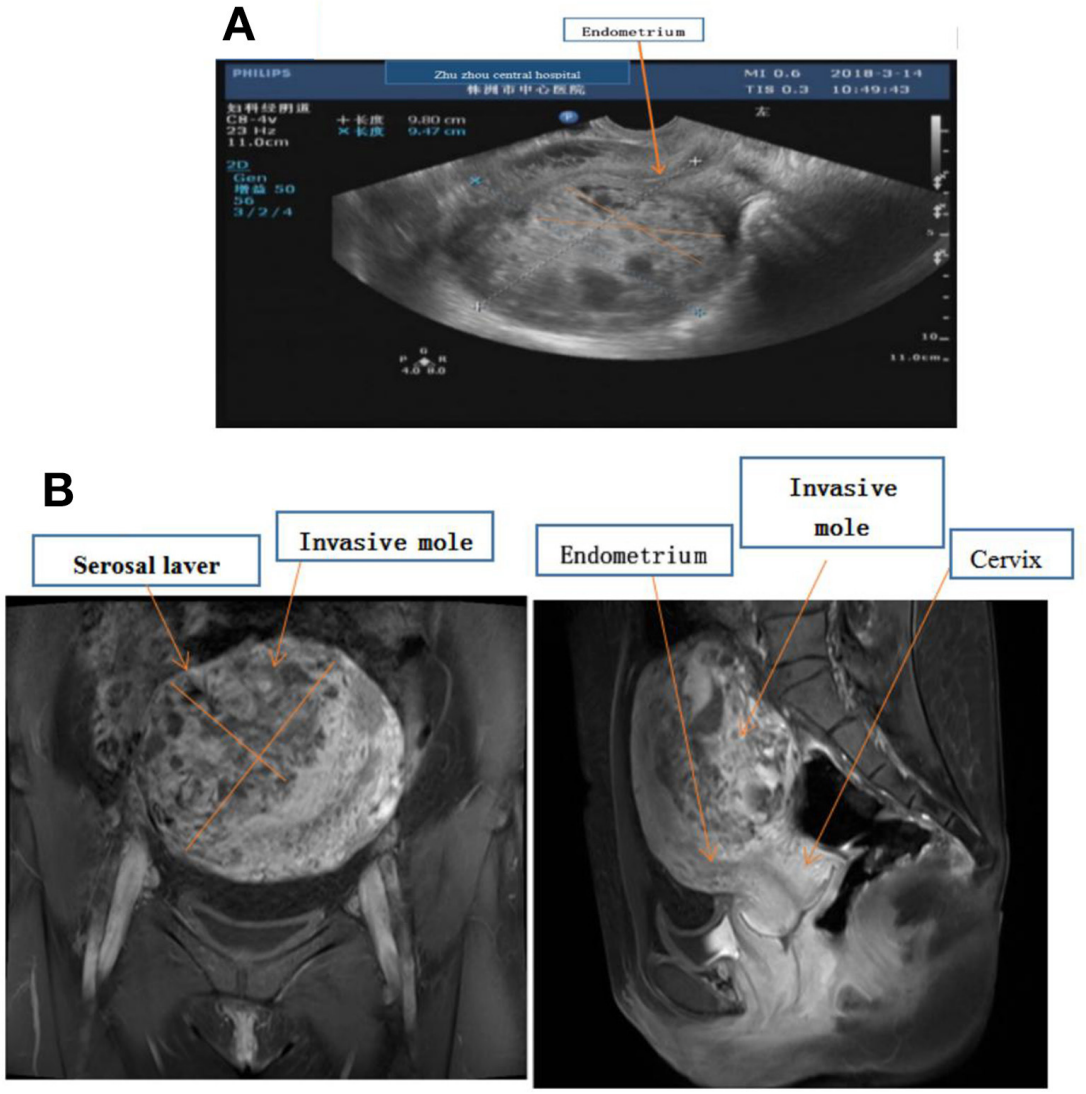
(A) Ultrasound examination showing fullness of the uterus, about 98 × 95 × 106 mm, the uterine cavity moved forward, and the endometrial thickness was 4 mm. There was about 85 × 70 × 97 mm hyperechoic masses in the muscular layer of the posterior wall, and the echoes were disordered and uneven. (B) MR imaging showing enlarged, uterus 100 × 96 × 105 mm, and there was a mass in the myometrium with the size about 12.3 × 8.0 cm, which invaded the serosal laver but not endometrium.
